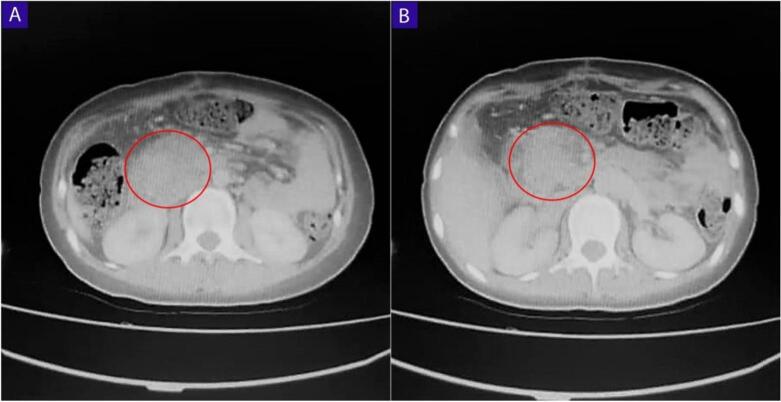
Abdominal CT scan (A and B) axial sections, a well-defined cyst (red circle), measuring 10 cm in the right hypochondriac fossa adhering to the head of the pancreas. (For interpretation of the references to color in this figure legend, the reader is referred to the web version of this article.)
